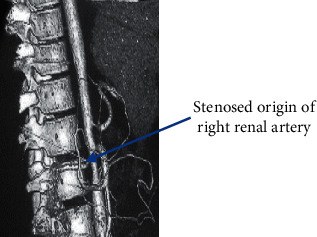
CTA showing the stenosed origin of the right renal artery.
